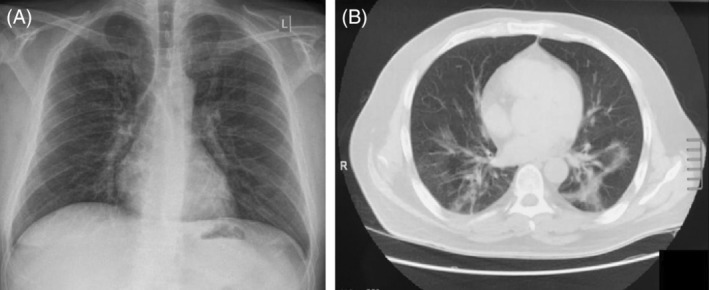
(A) Posteroanterior chest x‐ray shows bilateral ill‐defined linear opacities, mainly in the lower lung fields, L: left. (B) High‐resolution chest computed tomography shows ground‐glass opacities in the lower lung lobes, r: right
